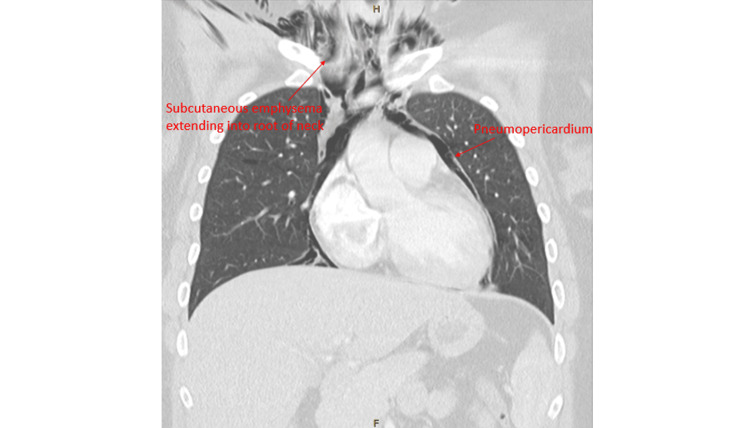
Coronal view of CT chest demonstrating significant pneumopericardium, pneumomediastinum, and subcutaneous emphysema extending to the root of the neck.
